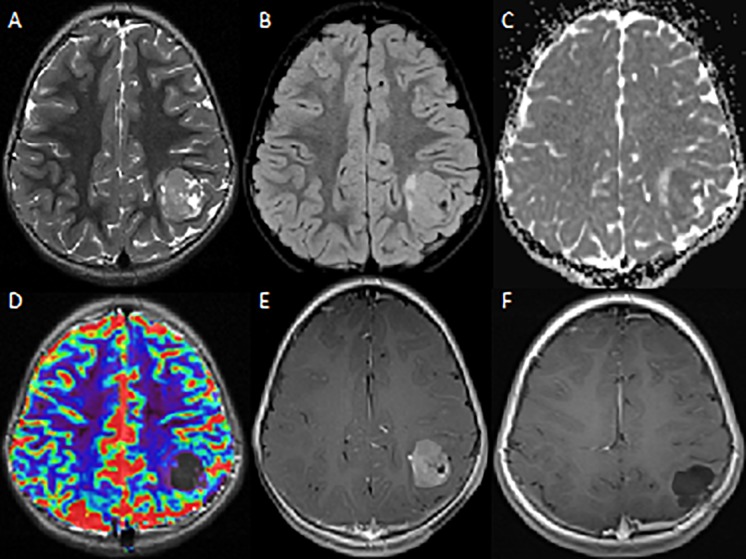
MRI – Axial images. (A) T2W image shows a heterogeneous mass in the left parietal lobe with characteristic multicystic bubbly appearance. (B,C) There are mild peritumoral edema on FLAIR image and diffusion restriction on ADC. (D) No sign of hyperperfusion on dynamic susceptibility-weighted MRI. (E) The T1W post-contrast image shows strong contrast-enhancement of the lesion. (F) Post-contrast-enhanced cerebral MRI performed 20 months after surgery shows no evidence of recurrent/residual disease.
